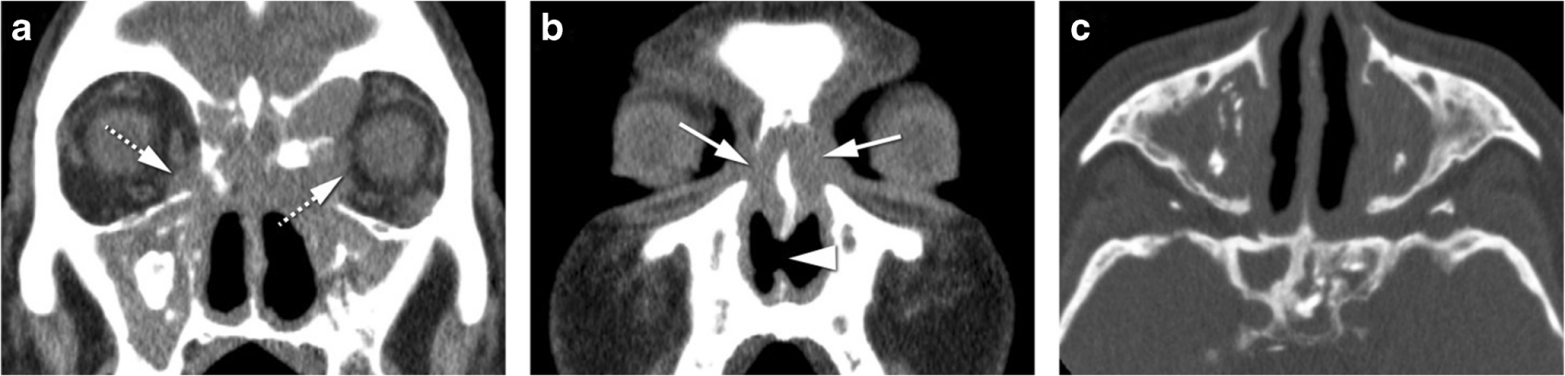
67-year-old male with granulomatosis with polyangiitis involving the orbit, nose, and sinuses. Coronals (a, b) and axial (c) CT: chronic pansinusitis with sclerosis of the bony walls and intrasinusal calcifications coexisting with nasal septum (arrowhead) and lamina papiracea (arrows) erosions. Bilateral orbital involvement in the extraconal compartment (dashed arrows)
